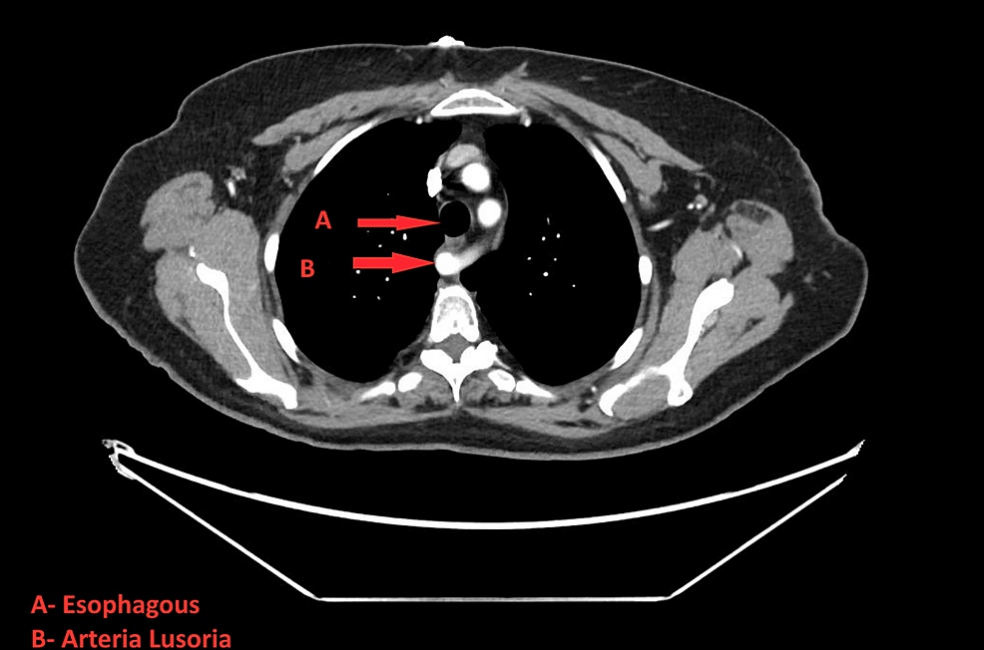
Computed tomography angiogram of the chest, abdomen, and pelvis The arrows above point to an aberrant right subclavian artery that lies posterior to the esophagus at the level of the upper thorax.
